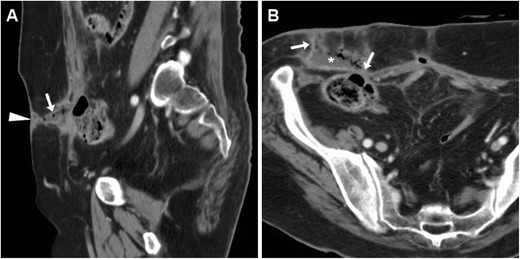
Postoperative ECF with subcutaneous abscess formation in a 71-year-old woman, following resection of sigmoid colon diverticulitis, complicated by anastomotic dehiscence which required re-laparotomy, ileal resection, and temporary ileostomy. CT diagnosed communication (arrows) between the cecum and an abscess (*) located just superficially to the ventral abdominal muscles, draining to the EFO (arrowhead in A). Surgical treatment included ileocecal resection and abscess toilette
